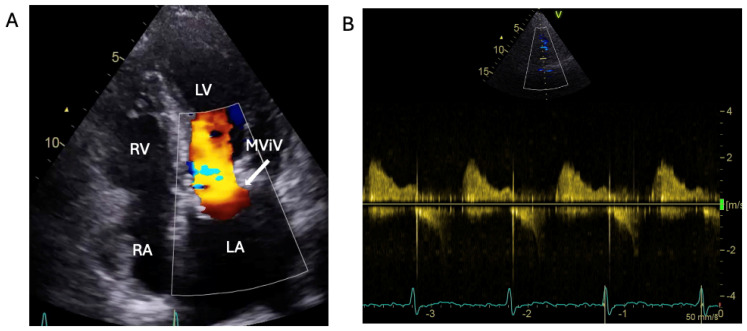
Transthoracic echocardiography colour Doppler and CW velocity after transcutaneous MViV intervention. (A): Colour Doppler flow of apical 4-chamber view of transthoracic echocardiography after percutaneous mitral valve-in-valve implantation (MViV). (B): Continuous Doppler velocity across MViV showing no significant inflow gradient. RV: right ventricle; LV: left ventricle; RA: right atrium; LA: left atrium.
